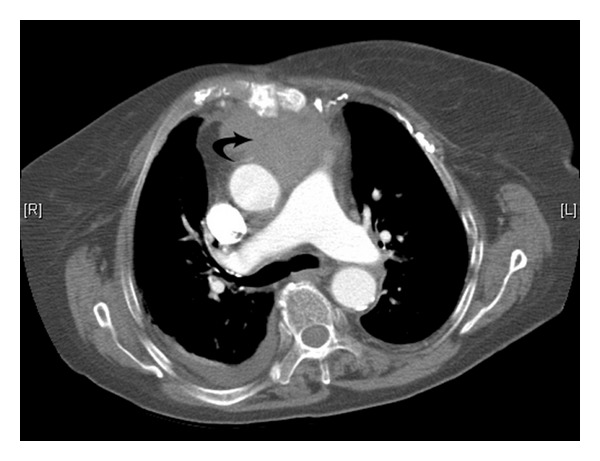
Axial slice from contrast-enhanced CT study depicting sternal osteomyelitis and a large related mediastinal collection (black curved arrow). The collection has higher density than that of water in keeping with pus and/or a degree of haemorrhage.
